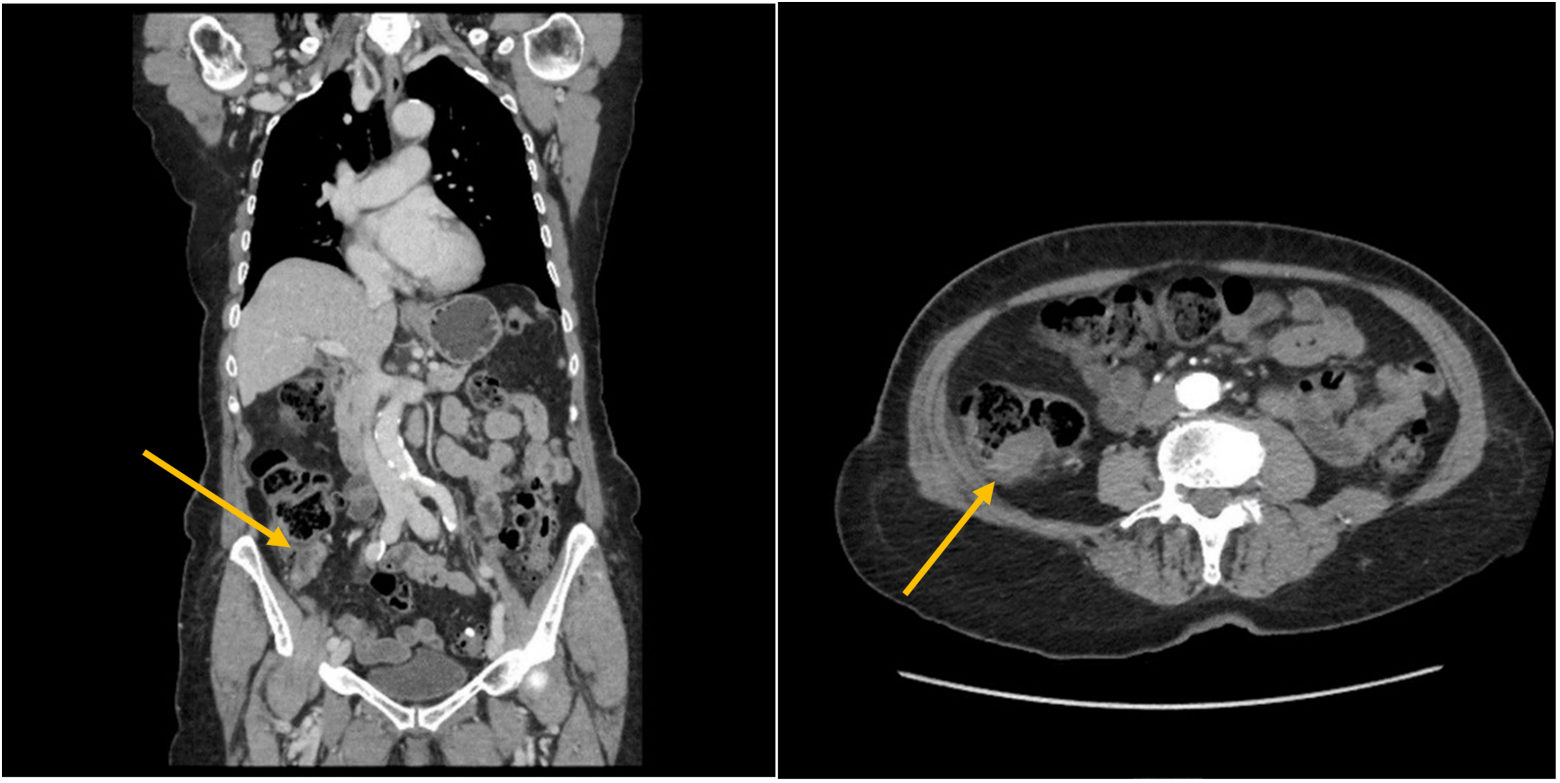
Abdominal CT scan view of a parietal tumor in the ascending colon without lymphadenomegaly or other pertinent abdominal alterations.
